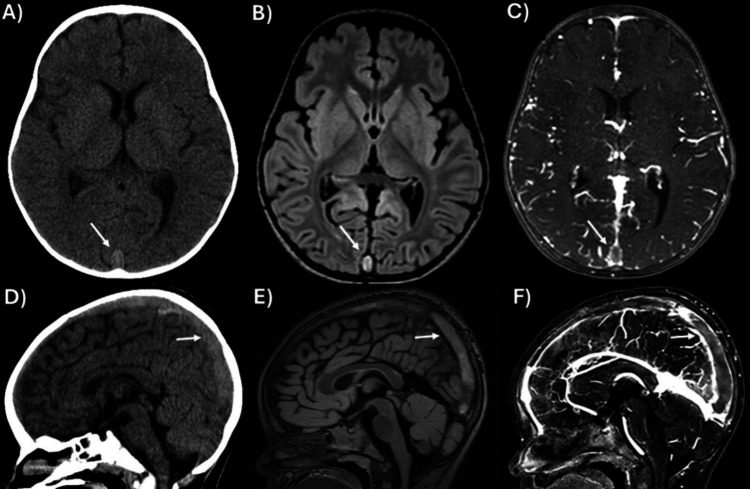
Cerebral Imaging at Presentation (A) Axial non-contrast CT shows a heterogeneous attenuated superior sagittal sinus (white arrow). (B) Axial FLAIR (Fluid Attenuated Inversion Recovery) shows thrombosis of the superior sagittal sinus (white arrow). (C) Axial magnetic resonance venography (MRV) shows thrombosis of the superior sagittal sinus (white arrows). (D) Sagittal non-contrast CT shows a heterogeneous attenuated superior sagittal sinus (white arrow). (E) Sagittal FLAIR shows thrombosis of the superior sagittal sinus (white arrow). (F) Sagittal MRV shows thrombosis of the cortical veins and the superior sagittal sinus.
